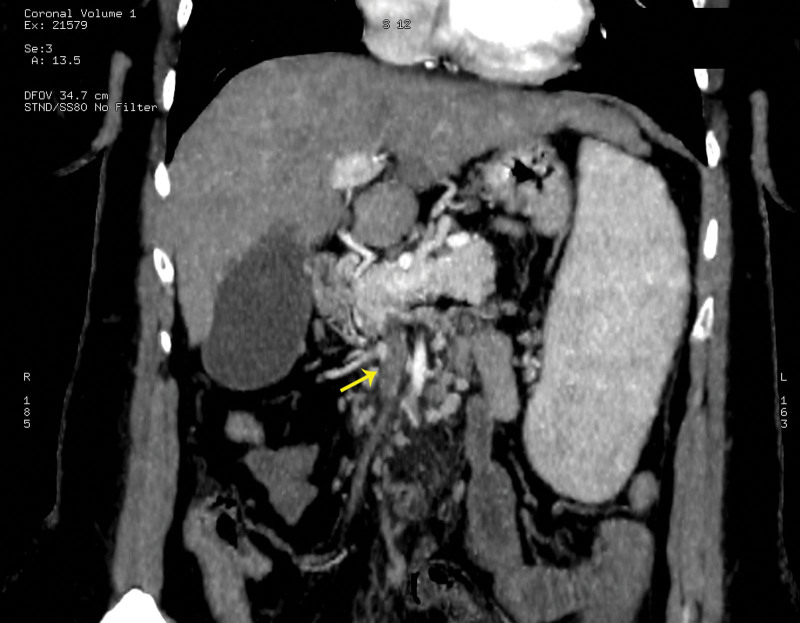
Intraluminal filling defect (yellow arrow) in the superior mesenteric vein on contrast-enhanced CT scan. CT = computed tomography.
